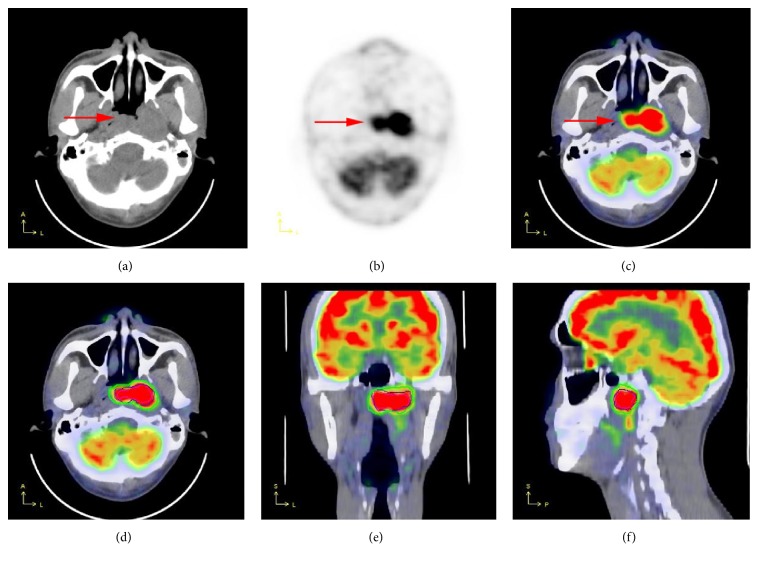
A 59-year-old male with NPC after comprehensive therapy. CT (a), PET (b), and fused (c) images show a mass lesion in nasopharyngeal posterior wall and left lateral wall (red arrow). Results of segmentation using the four described thresholds are shown on transaxial (d), coronal (e), and sagittal views (f). The light red, purple, blue, and green areas were segmented using SUVmax = 2.5, Th20, Th40, and Th_bgd_ thresholds, respectively.
